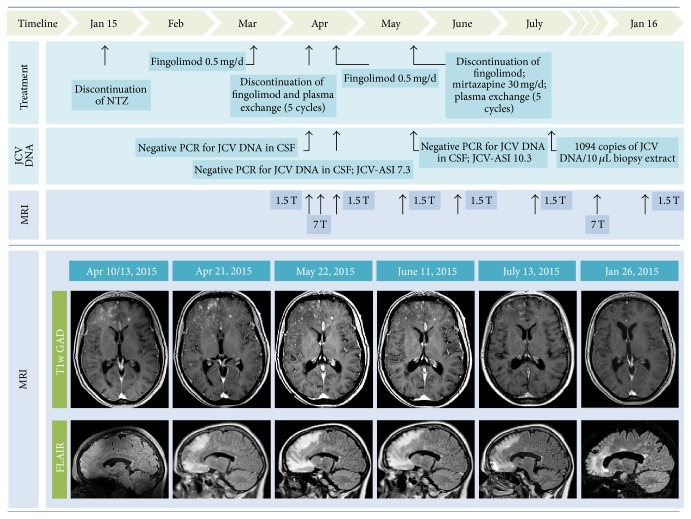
Overview. Survey of treatment decisions and laboratory and MRI findings of the patient under discussion. In detail, 1.5 T T1 weighted Gadolinium enhanced (T1W GAD) and 1.5 T fluid attenuated inversion recovery (FLAIR) images are presented.
